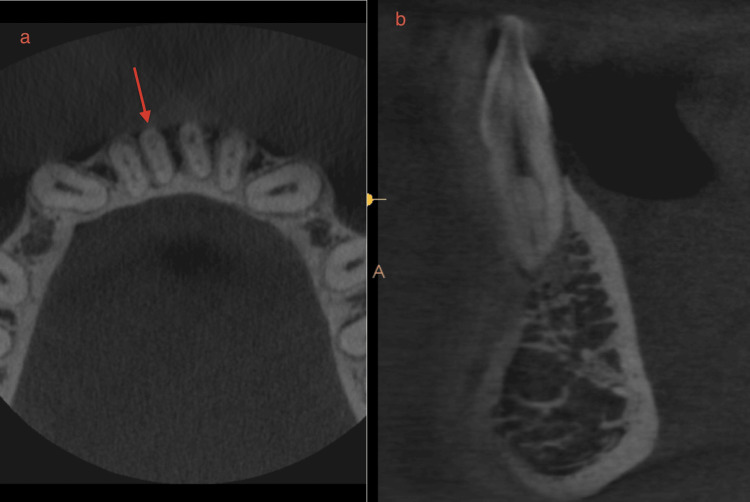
CBCT showing three canals or Ahmed 1-3-1 configuration. (a) Axial section. (b) Sagittal section. CBCT - Cone beam computed tomography
